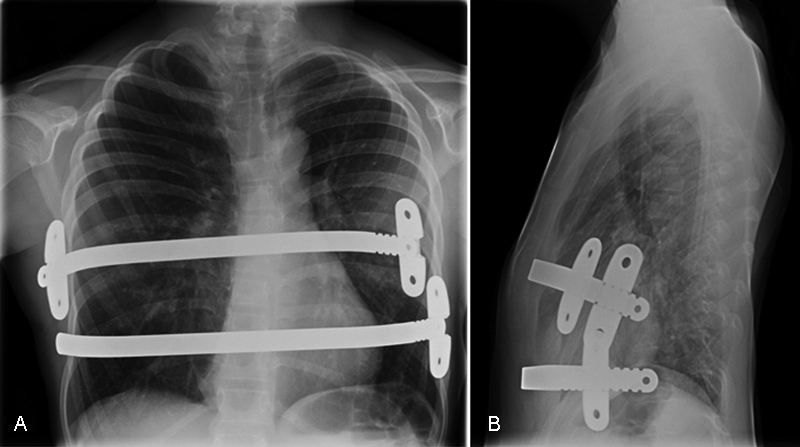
Postoperative results X-ray. (
